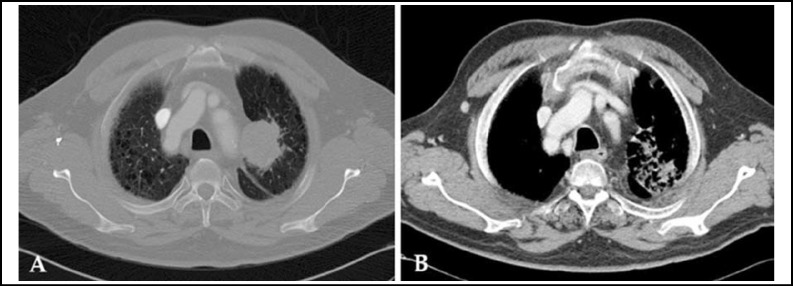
Chest computed tomography findings
